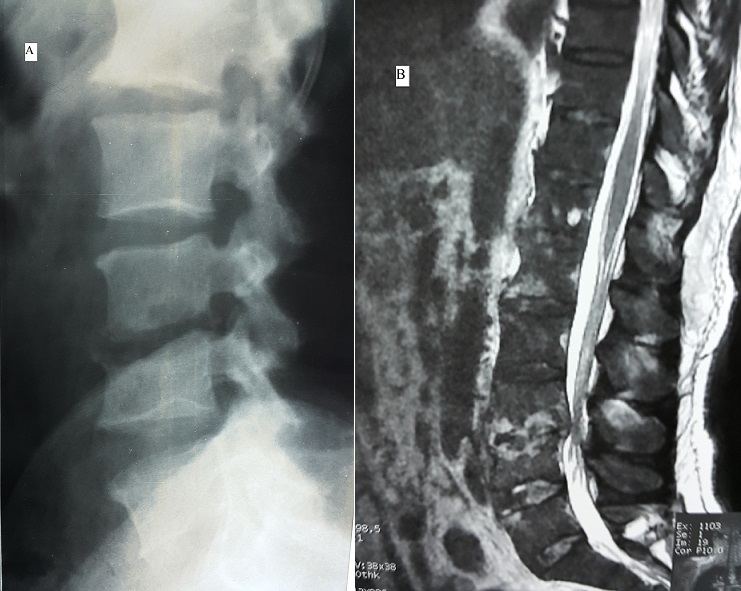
(A) radiographie rachis lombaire de profil montrant le pincement discal en L3-L4 avec érosion des plateaux vertébraux; (B)IRM lombaire, coupe sagittale en séquence pondérée T2 montrant l'hyper signal des plateaux vertébraux en L3-L4avec perte de la limite vertèbre disque
